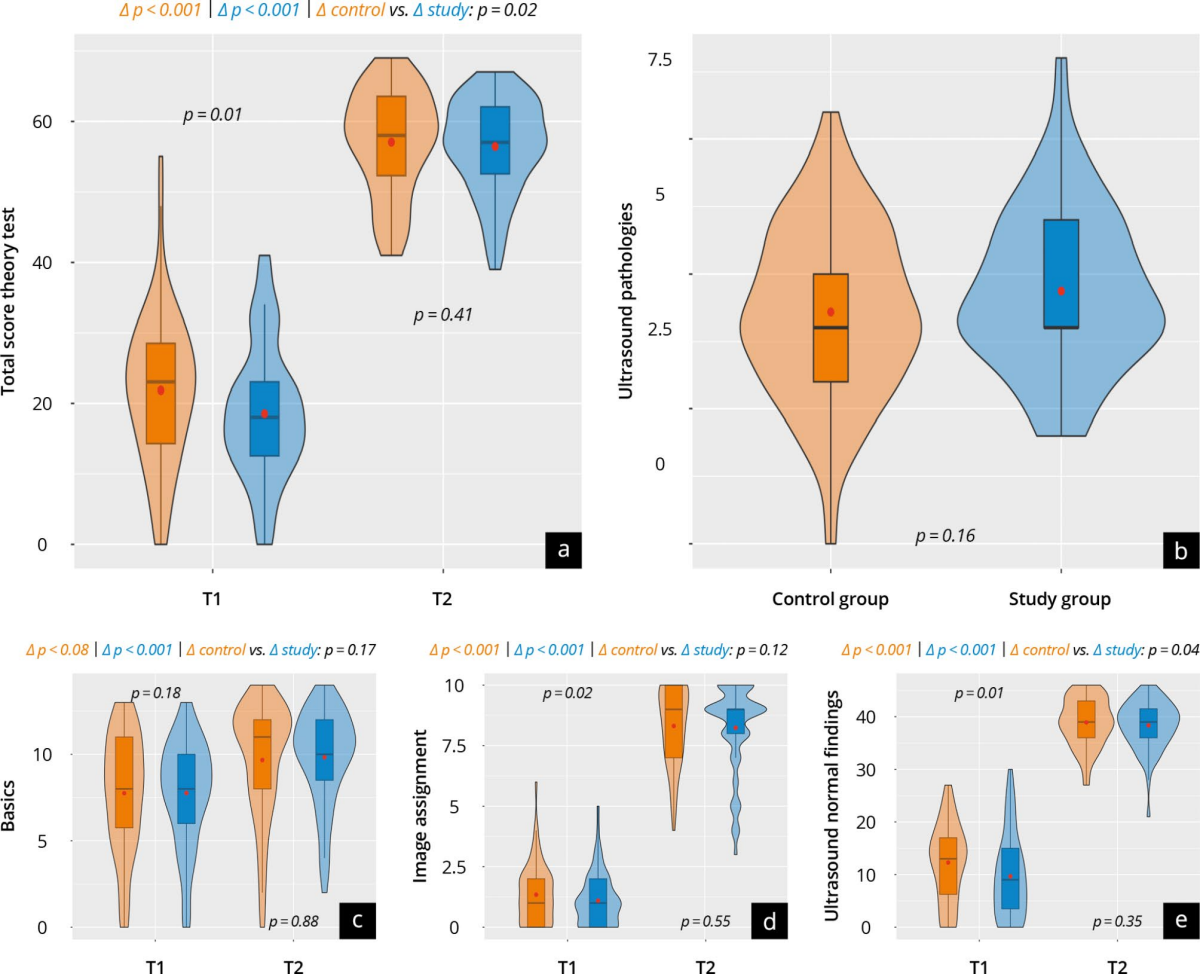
Results of the theory test at T_1_ and T_2b_ with the control group represented in orange and the study group in blue. (a) The total score; (b) the subcategories ultrasound pathologies; (c) basics; (d) image assignment; (e) ultrasound normal findings
